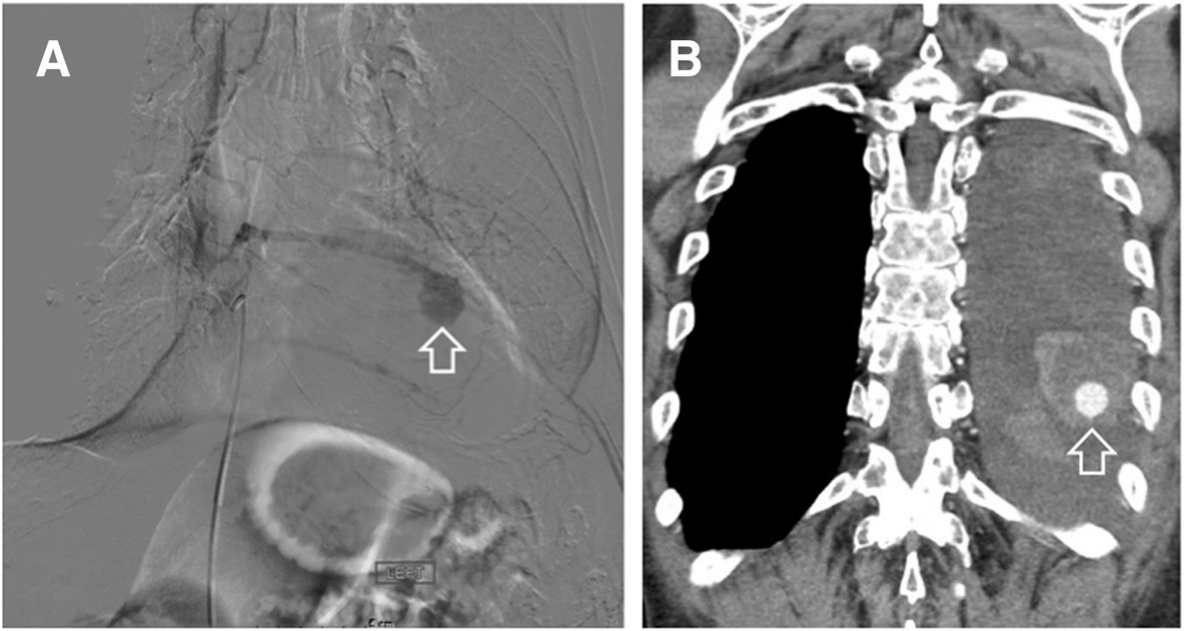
a Selective digital subtraction angiogram of the intercostal artery pseudoaneurysm (arrow). b IV contrast-enhanced computed tomography (CT) image, coronal projection, depicting the pseudoaneurysm (arrow)
